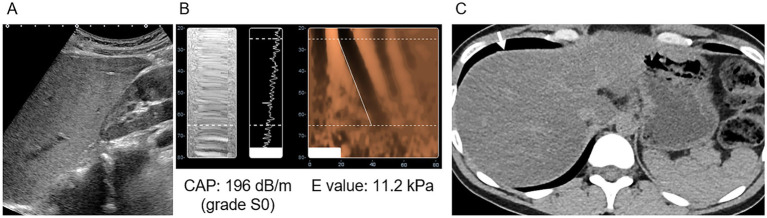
Imaging findings at the onset of acute fatty liver of pregnancy. (A) Abdominal ultrasonography showing mildly increased hepatorenal contrast. (B) Representative images obtained by vibration-controlled transient elastography (FibroScan®), showing the controlled attenuation parameter (CAP) and liver stiffness (E value). (C) Non-contrast abdominal computed tomography demonstrating diffusely decreased hepatic parenchymal attenuation, with a CT value of 23.0 Hounsfield units (HU; white arrow).
